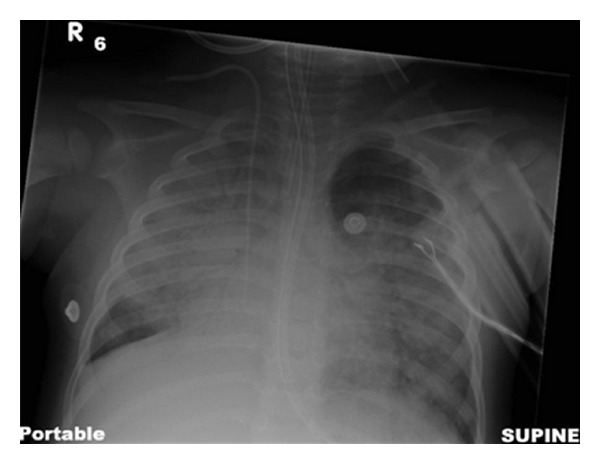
Initial chest radiograph prior to ECMO initiation.
